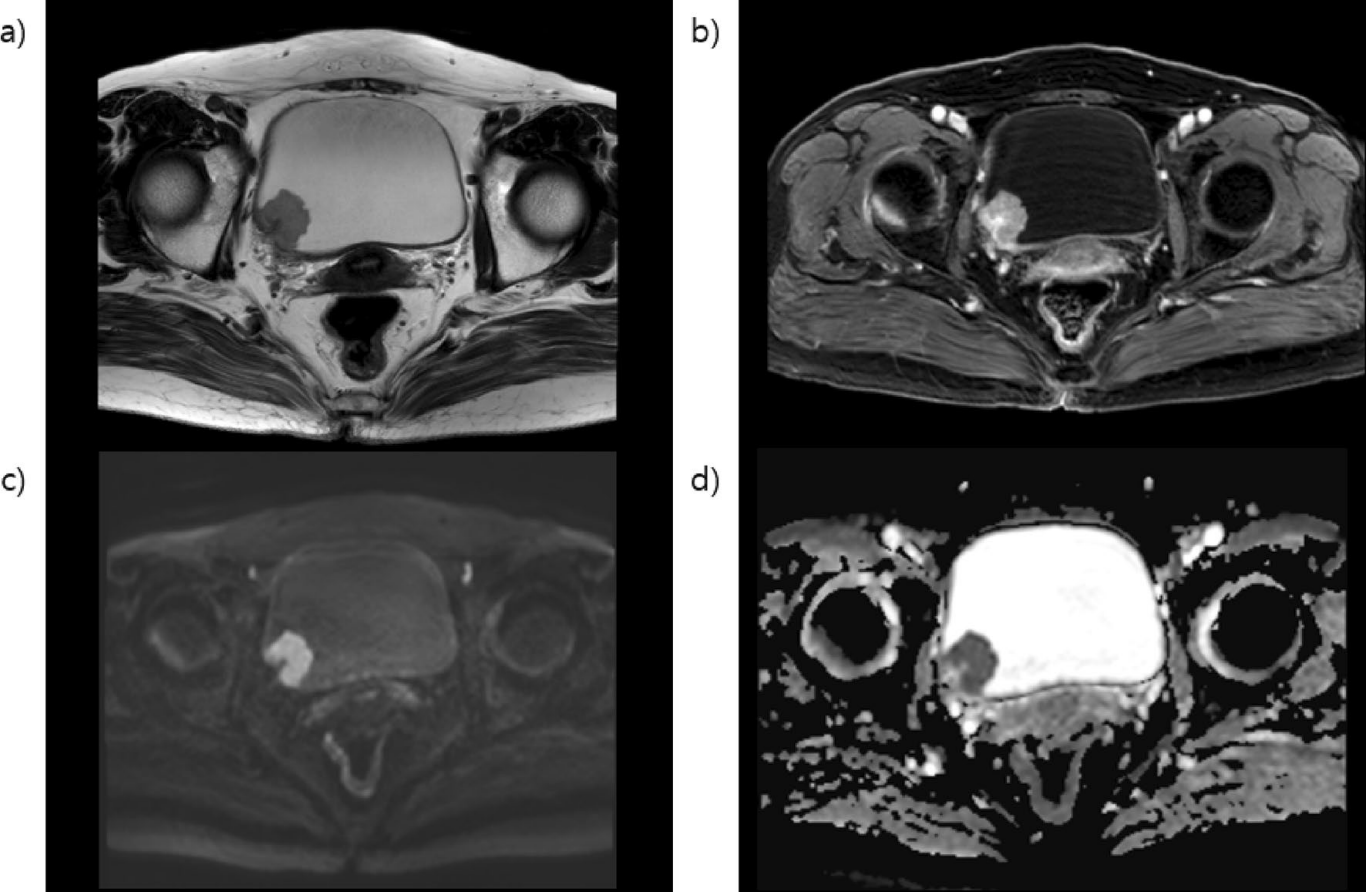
Magnetic resonance images of muscle-invasive bladder cancer (a) T2-weighted image in the right posterolateral aspect of the bladder wall showing tumor with stalk without high SI thickened inner layer with no clear disruption of low SI muscularis propria. (b) Early enhancement of muscularis propria with early enhancement of inner layer but with no clear disruption of low SI muscularis propria. (c) Diffusion-weighted imaging (DWI) native image and (d) apparent diffusion coefficient (ADC) map showing high SI tumor on DWI and low SI tumor on ADC extending focally to muscularis propria. The muscle layer invasion of the tumor is likely.
